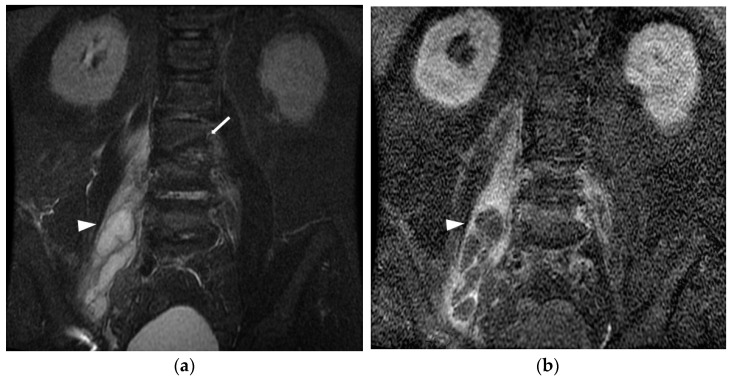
Pyogenic spondylodiscitis complicating iliopsoas abscess in a 58-year-old male. (a) Magnetic resonance (MR) image of L-S spine of coronal short T1 inversion recovery (STIR) sequence showed cortical discontinuities and erosions of endplates (arrow) of L2, L3 vertebras with hyperintense of right iliopsoas muscle associated with intramuscular loculated fluid collections (arrowhead). (b) Contrast-enhanced MRI T1 weighted image (WI) with fat suppression showed prominent rim enhancement of loculated fluid collection in the right iliopsoas muscle, which was compatible with intramuscular abscess of the right iliopsoas muscle (arrowhead).
